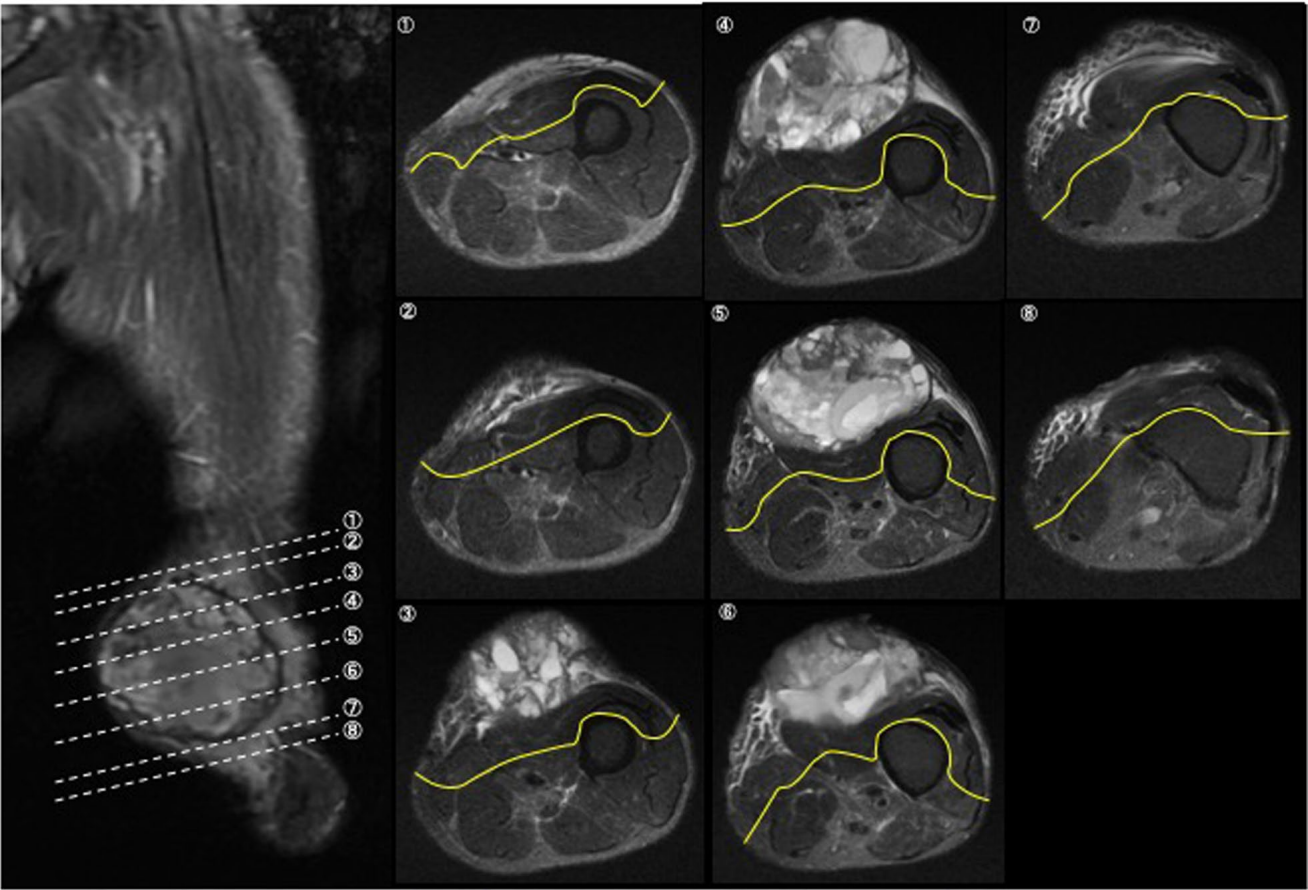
Procedure for wide resection of the femur. Solid (yellow) lines are resection lines on T2-weighted images. En bloc wide resection of the sarcoma included the quadriceps femoris and skin. Tumours were severed 20 mm proximally, 20 mm distally, and 20 mm dorsally and included the reactive layer. The rectus femoris, vastus medialis, sartorius, and vastus intermedius were separated at the tumour’s maximally bulging region. A portion of the vastus lateralis was excised. 1–8 (lower left) shows the levels at which the resections were performed
